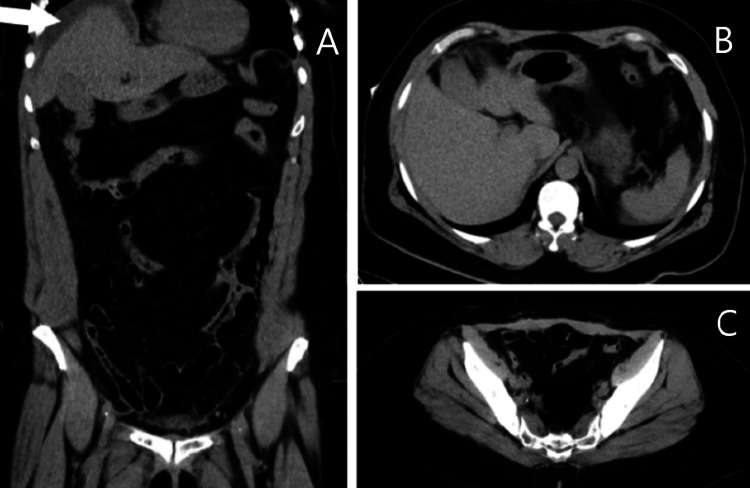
Follow-up CT scan after catheterization Abdominal CT after catheterization, showing a reduction in the ascitic fluid (marked by the white arrow). Sub-image A shows the coronal plane. Sub-images B and C show the axial plane.
